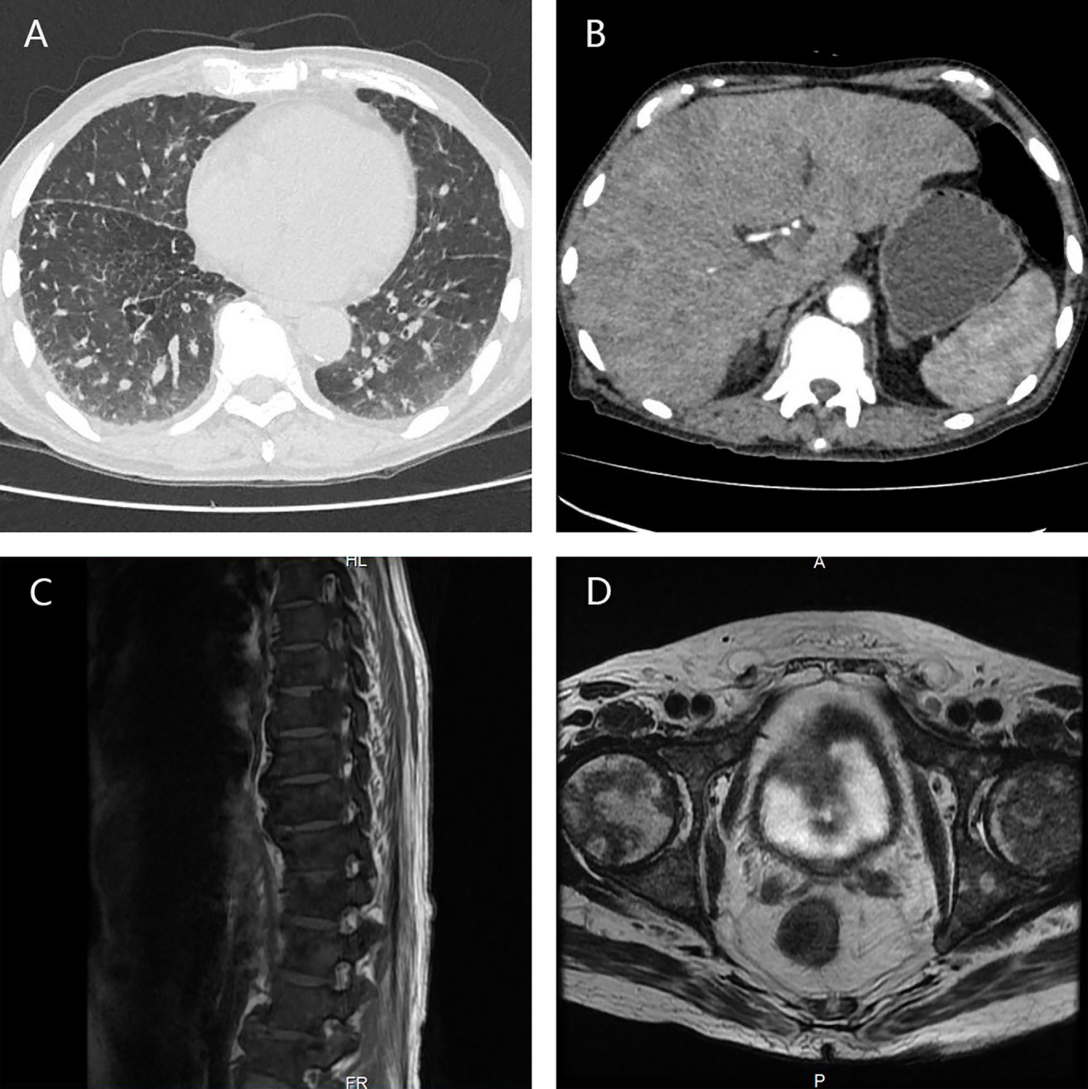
CT/MRI scan showed widespread metastases in the lungs (A), liver, bilateral adrenals (B), thoracic and lumbar vertebrae (C), and pelvis bones (D).
